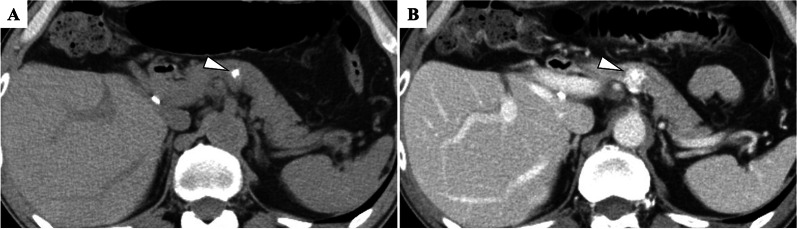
Computed tomography shows a 4-mm calcification (A) and a 10-mm contrast-enhanced mass (B) in the pancreatic body
